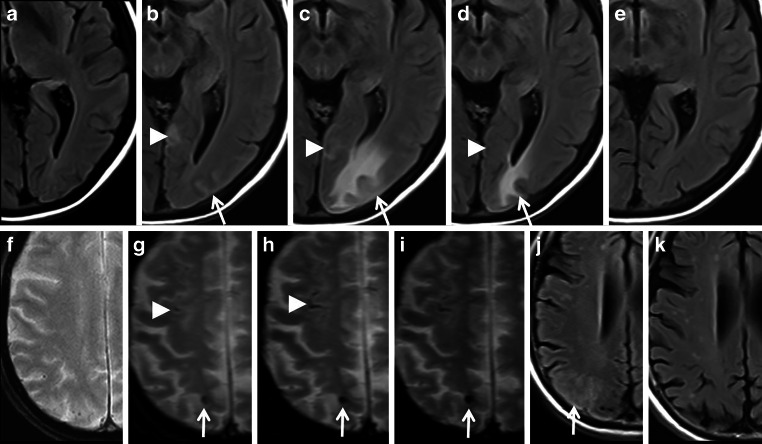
Amyloid related imaging abnormalities (ARIA). a–e fluid attenuated inversion recovery [FLAIR] images ax. showing encephalopathic type of ARIA (ARIA—E) in a 54-year-old man treated with aducanumab, weeks 14 (a), 30 (b), 34 (c), 38 (d) 40 (e) after treatment initiation; sulcal effusions (b–d, *arrowhead*) and additional hyperintense lesion in the occipital lobe (b–d, *arrow*), which completely resolved at week 40. T2* WI ax. (f–i) and FLAIR ax. (j,k) demonstrating hemorrhagic type of ARIA (ARIA-H) and ARIA‑E in a 68-year-old woman treated with aducanumab at baseline (f), weeks 14 (g), 18 (h,j), 20 (i) and 94 (k)
